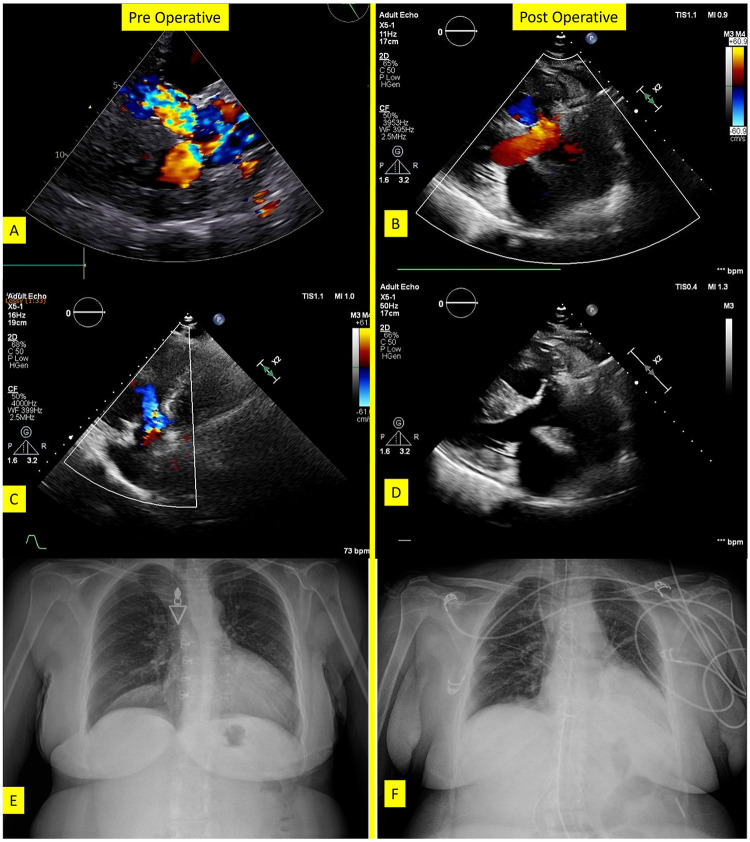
(A,C) Pre-operative VSD showing mixing. (B,D) Postoperative closed VSD with a patch and no residual mixing. (E) pre-operative chest x-ray (CXR) showing boot-shaped heart; (F) post-operative CXR. The linear radiopaque densities projected over the sternum in section E are external clothing-related artifacts and do not represent sternal wires. The patient had no history of prior median sternotomy or cardiac surgery.
